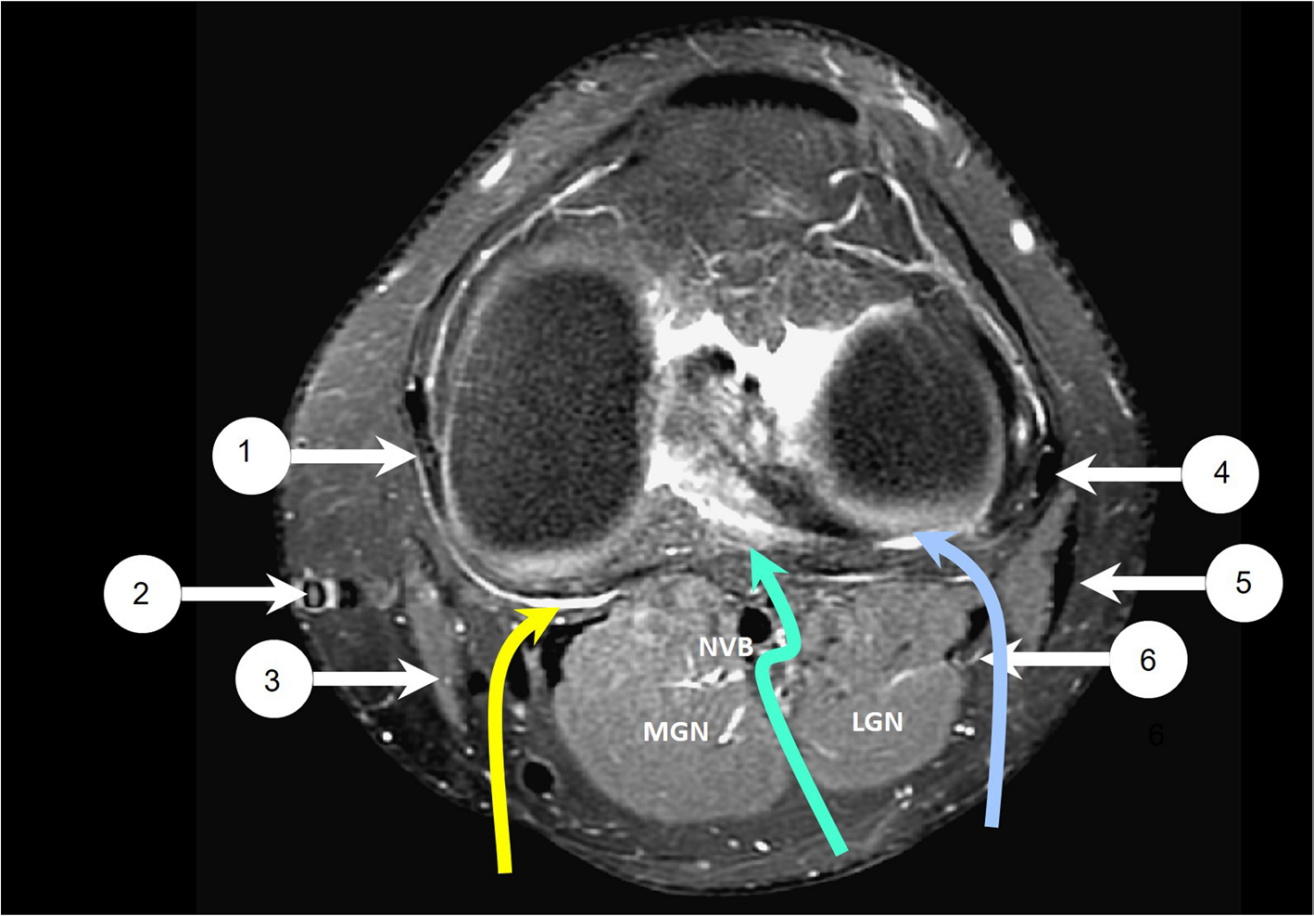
Left knee MRI of a healthy 21-year-old man. *Proton density* with fat saturation sequences axial view 1. Medial collateral ligament; 2. Great saphenous vein; 3. Sartorius and gracilis muscle; 4. Lateral collateral ligament; 5. Biceps femoris tendon and muscle; 6. Common peroneal nerve. Yellow arrow: PM approach; Green arrow: DP approach; Blue arrow: PL approach. MGN: Gastrocnemius muscle medial head; NVB: Neurovascular bundle; LGN: Gastrocnemius muscle lateral head.
